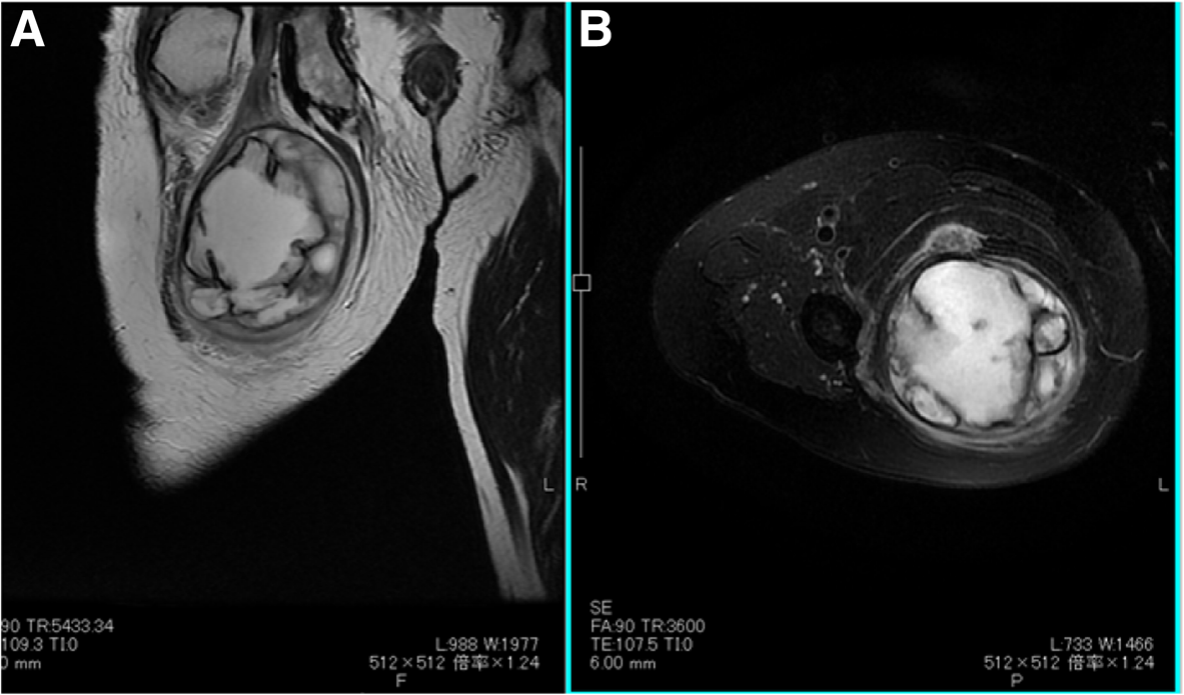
a, b T2-weighted (a) and T2-weighted fat-suppressed (b) MR images of mass showing the enlargement and homogenous appearance of contents with high signal intensities, indicating cystic degeneration
